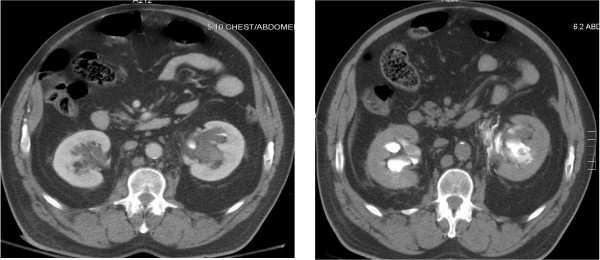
63 year old male. Contrast enhanced (left) and delayed (right) CT scans demonstrating stranding and fluid around the mid left ureter, and a left UPJ injury respectively.
